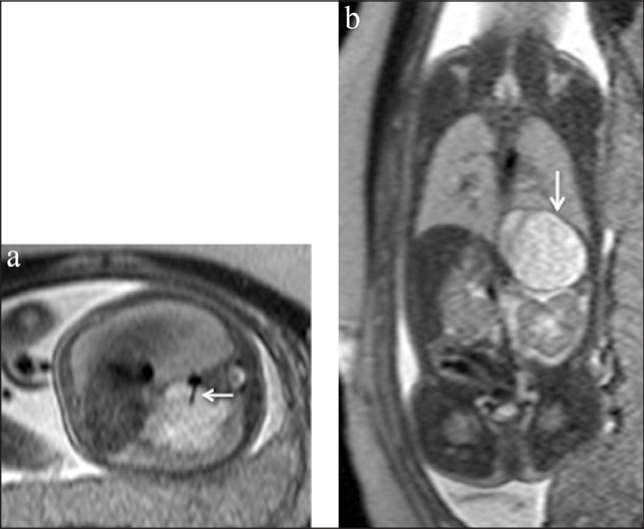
Intra diaphragmatic sequestration. Axial (a) and coronal (b) T2-weighted sequences on the thorax of a 27 week-old foetus showing a well circumscribed hyperintense lesion with a systemic feeding vessel (a, arrow) confirming the diagnosis of a sequestration. The hypointense capsule (b, arrow) is suggestive of an intradiaphragmatic lesion that was confirmed by surgery.
